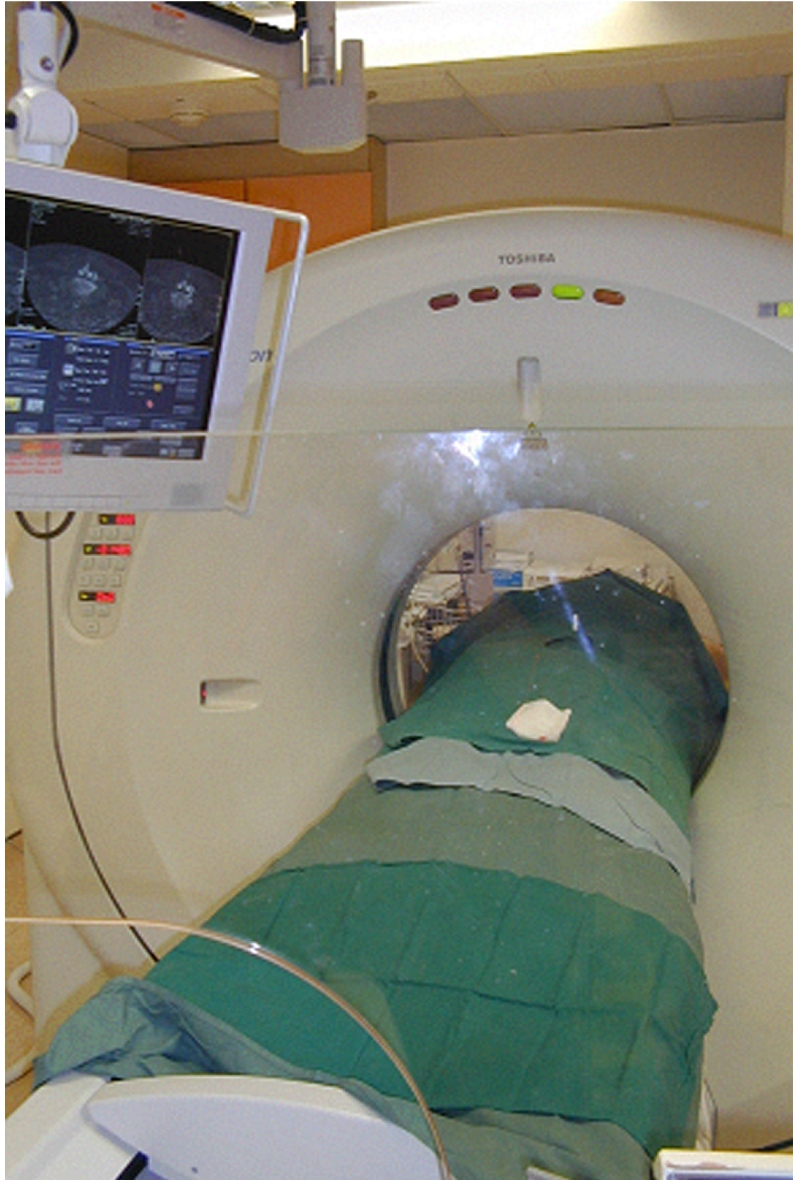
Photograph of a CT fluoroscopy unit. The radiologist stands behind the lead glass shield in the foreground and moves the CT couch-top either manually or via remote control. The CT images are observed on the monitor.
